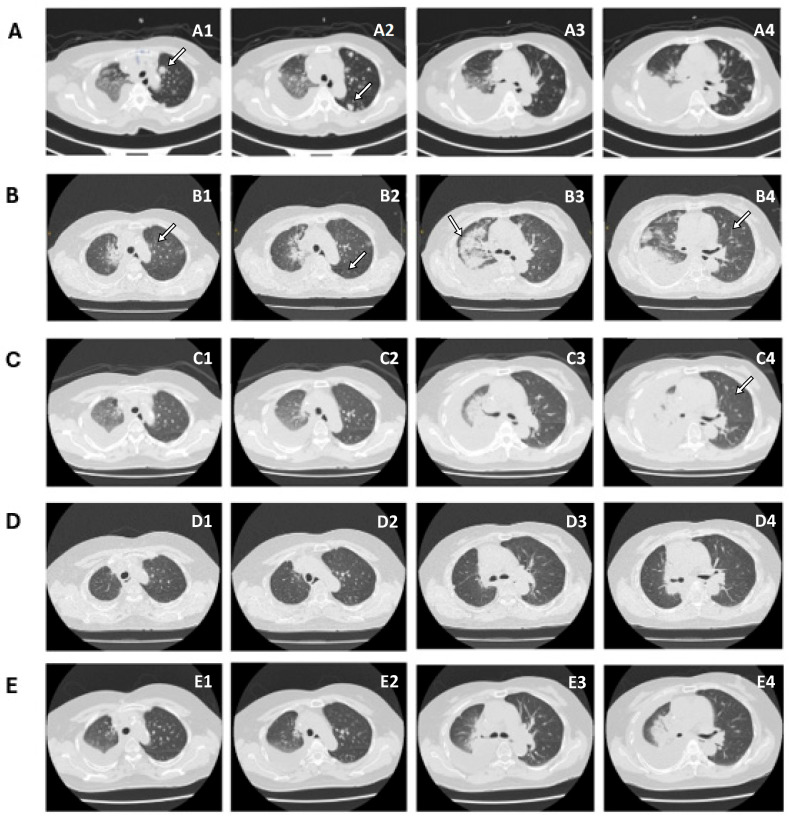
Serial follow-up CT imaging after entrectinib administration demonstrates improved outcomes. (A) CT imaging shows the initial diagnosis performed on 18 January 2024. (B) Compared with the prior PET-CT, a marked regression of the primary right lung tumor and left lung metastases can be observed, particularly evident when comparing A1 with B1 and A2 with B2. Residual mediastinal and right hilar lymphadenopathy (station 4R: 2.5 → 1.7 cm; station 7: >2 → 1.1 cm), as well as left lung metastases (largest in Left lower lung(LLL): 1.6 → 0.9 cm), appear smaller and fewer. Post-radiation pneumonitis and treatment-related changes are visible on the 22 April 2024 image B3. A further reduction in mediastinal lymph node burden is clearly appreciated when comparing images B4 and C4. (C) Bilateral pulmonary metastases (<1 cm) and metastatic mediastinal lymph nodes (largest: 1 × 1 cm in the left paratracheal area) are slightly smaller and fewer in number on 25 September 2024. (D) Metastatic lymph nodes in the bilateral mediastinum and bilateral lung metastases appear reduced in size and number on 12 December 2024. (E) Right lung cancer status post concurrent chemotherapy(CCRT), with partial regression, on 17 March 2025.
